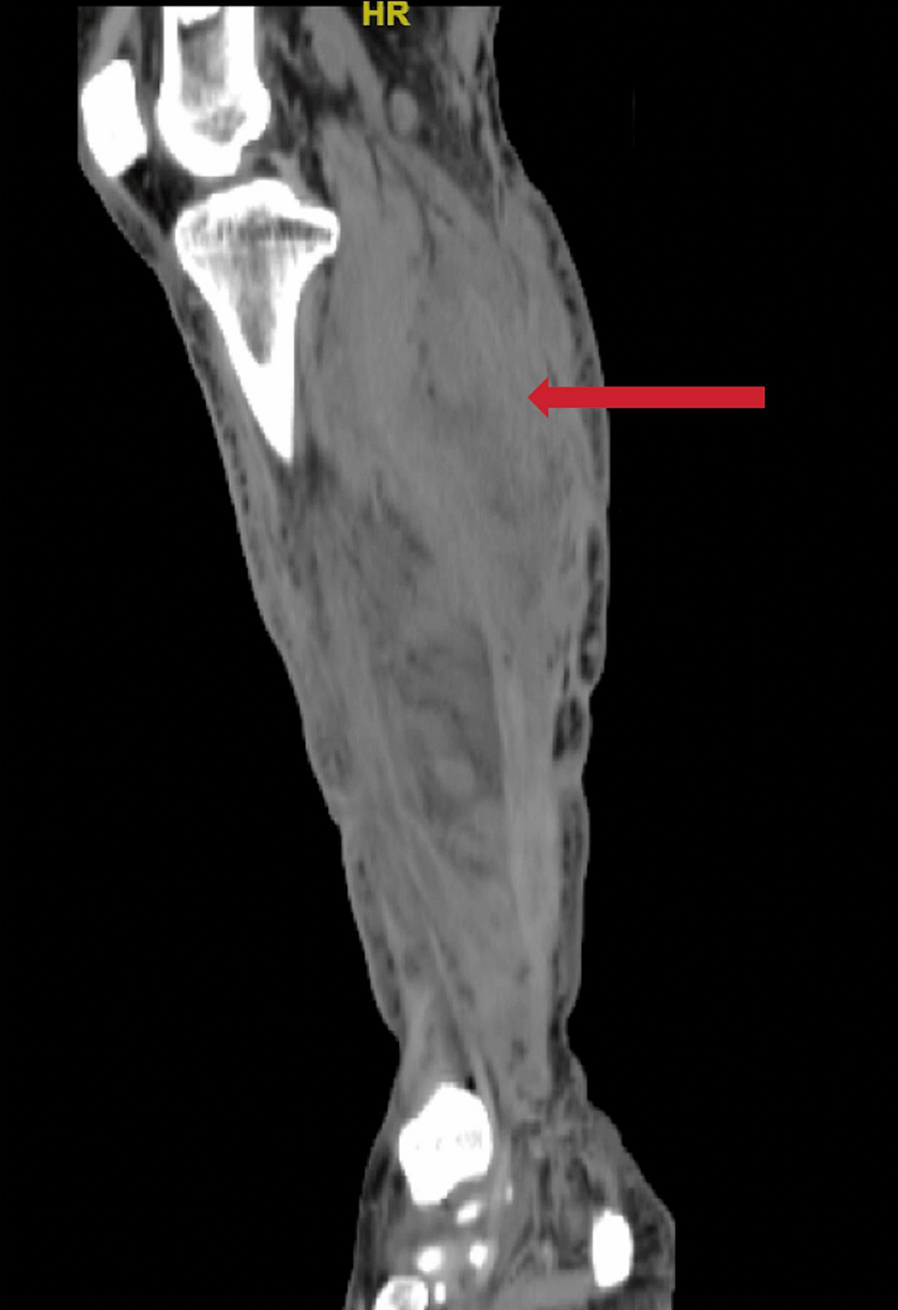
CT scan of the right distal lower extremity with diffuse subcutaneous fat stranding and a heterogeneous collection in the posteriomedial calf as indicated by the red arrow CT: Computed tomography
